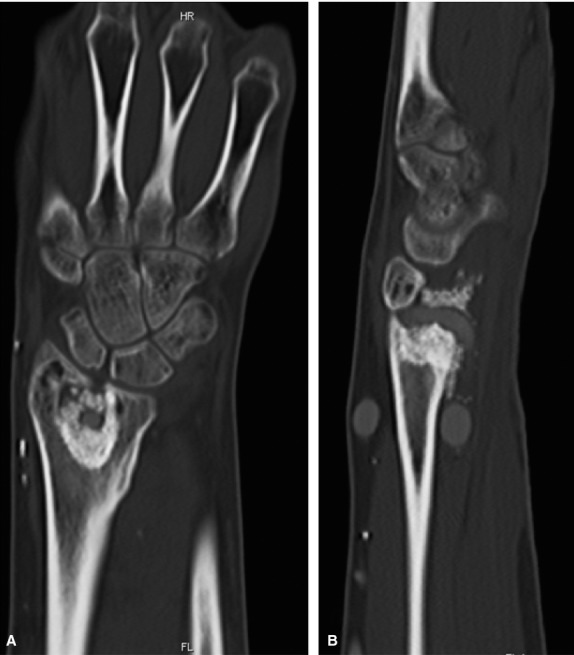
CT scan 14-month post-operative (case 2) demonstrating a completely healed bone defect in the distal radius. (A) Coronar reconstruction. (B) Sagittal reconstruction.
